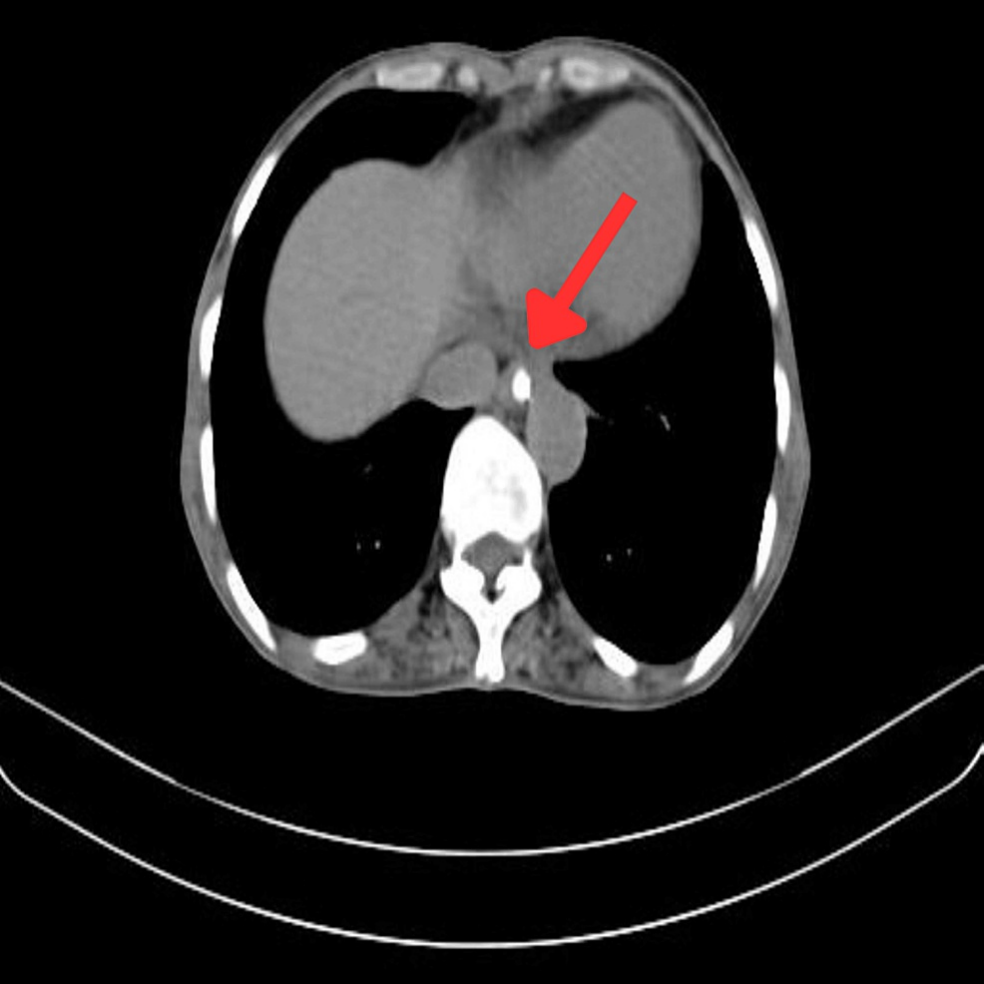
CECT thorax with the abdomen The red arrow points at the asymmetrical circumferential wall thickening of the esophagus. CECT, contrast-enhanced computed tomography
